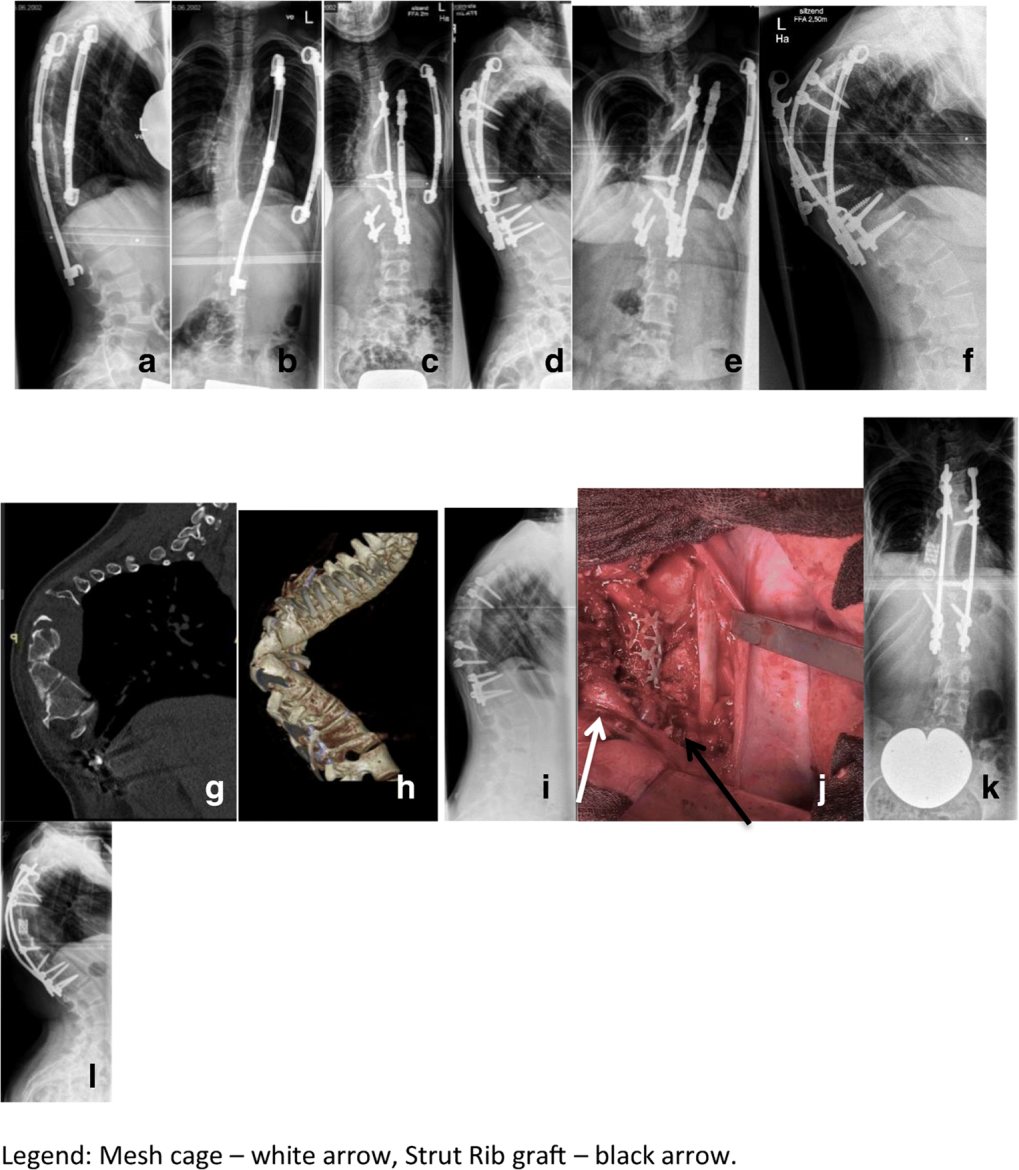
(Case 7) Eleven-year-old NF-1 patient with moderate dystrophic scoliosis (a–l) . The index procedure comprised a growth-preserving technique by means of vertical expandable titanium ribs (VEPTR). The curve was well-controlled and worsening was prevented for 4 years after initial surgery (a, b). During the pubertal growth spurt, significant curve progression occurred. An “in situ” posterior fusion combined with concave instrumentation was performed at age 13 (c, d). On further follow-up, the curve increased significantly due to the “modulation” process of dystrophic alterations (e, f). Note the severe dystrophic alterations and the complexity of the deformity seen on reconstruction CT scans (g, h). The patient developed an incomplete flaccid paraplegia of the lower extremities due to an increase of thoracic kyphosis resulting in anterior compression of the spinal cord. The treatment approach comprised of removal of instrumentation and halo-gravity traction for 4 weeks (h1) followed by posterior and anterior spinal fusion. A photo of the anterior procedure showing the intervertebral cages and the strut rib graft in place (j). Postoperative X-rays showing good deformity correction (k, l)
